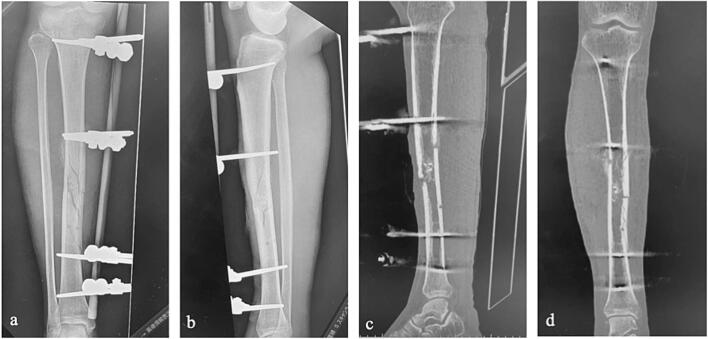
Radiological findings upon initial presentation. a. Anteroposterior plain radiograph. b. Lateral plain radiograph. c. Sagittal computed tomography image. d. Coronal computed tomography image.
